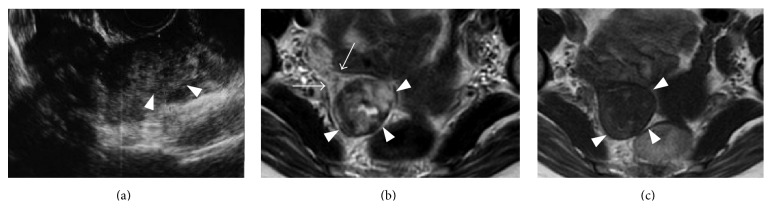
(a) Transvaginal ultrasonography reveals an echogenic mass (arrowhead) incarcerated to the right ovary and a normal endometrium. (b) Axial T2-weighted MR image shows a GS structure of heterogeneous high intensity (arrowhead), containing punctate foci of distinct low intensity. The GS is incarcerated to the right ovary, forming a “beak sign” (arrows). (c) Axial T1-weighted MR image showed GS structure (arrowhead) containing punctate foci of high intensity.
